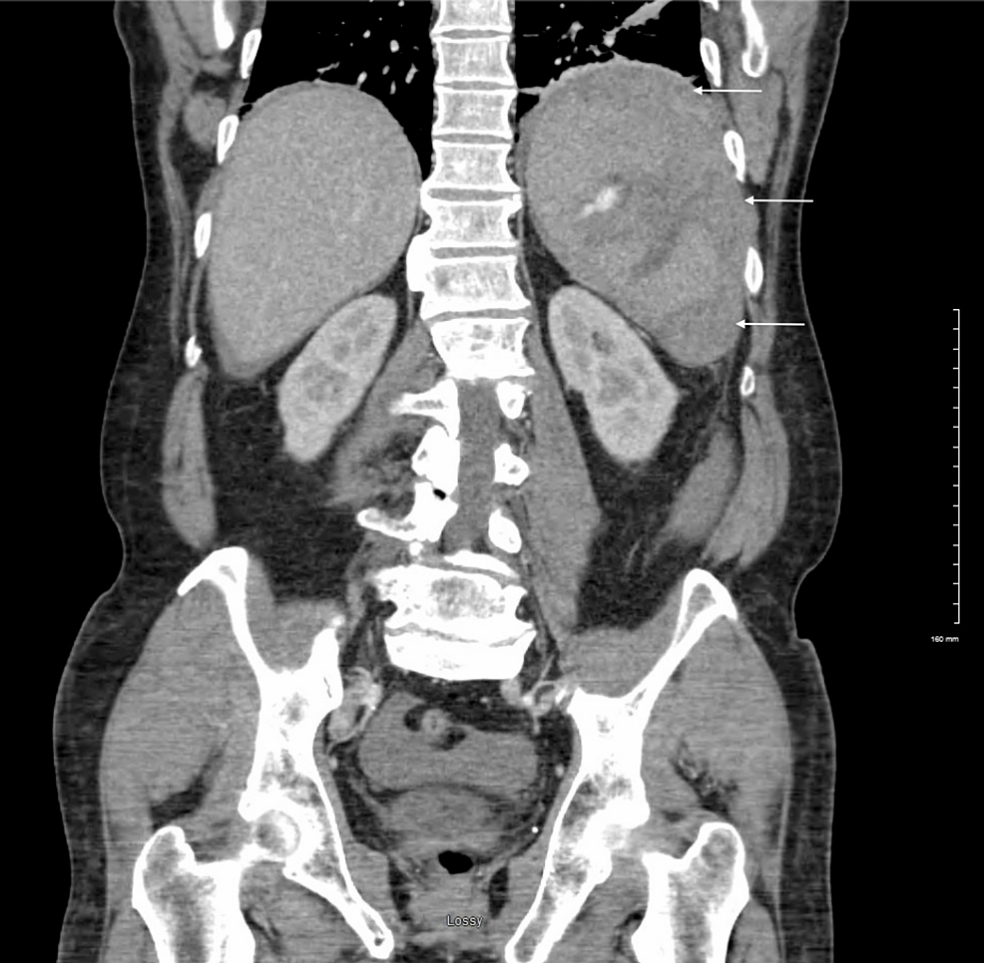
Coronal CT of the abdomen and pelvis with contrast showing a large area of hypodensity consistent with a splenic hematoma
